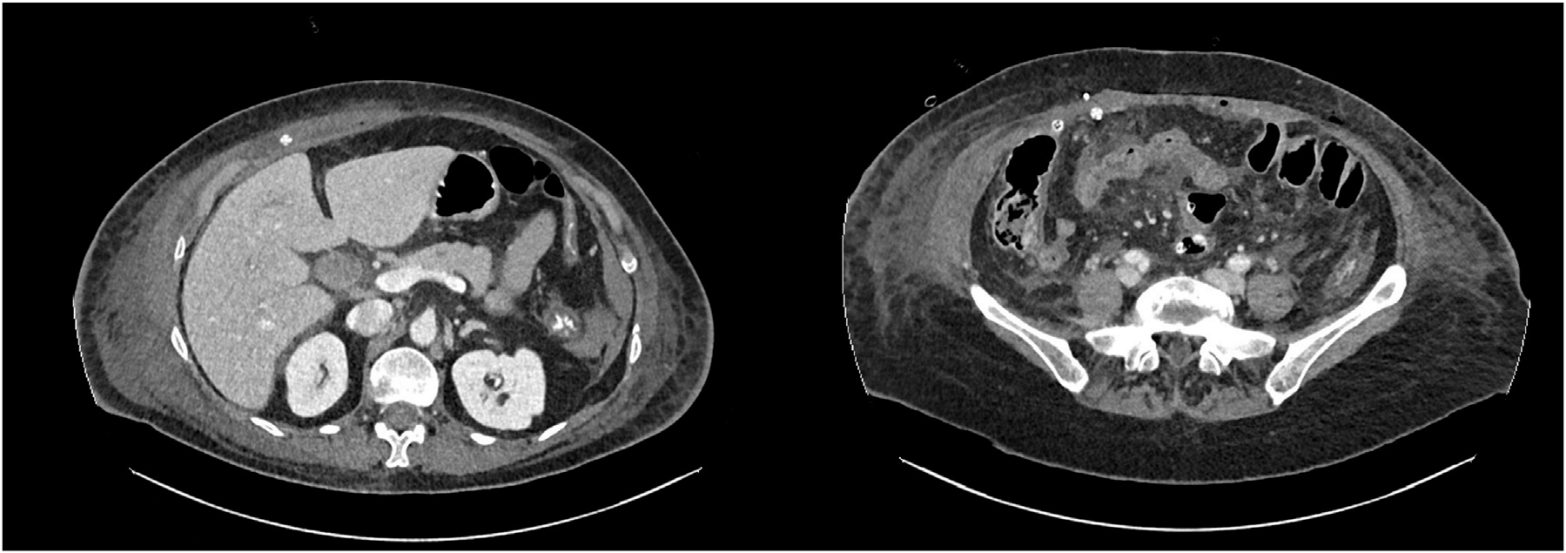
Postoperative CT scan with retromuscular and subcutaneous drainage above the mesh.
